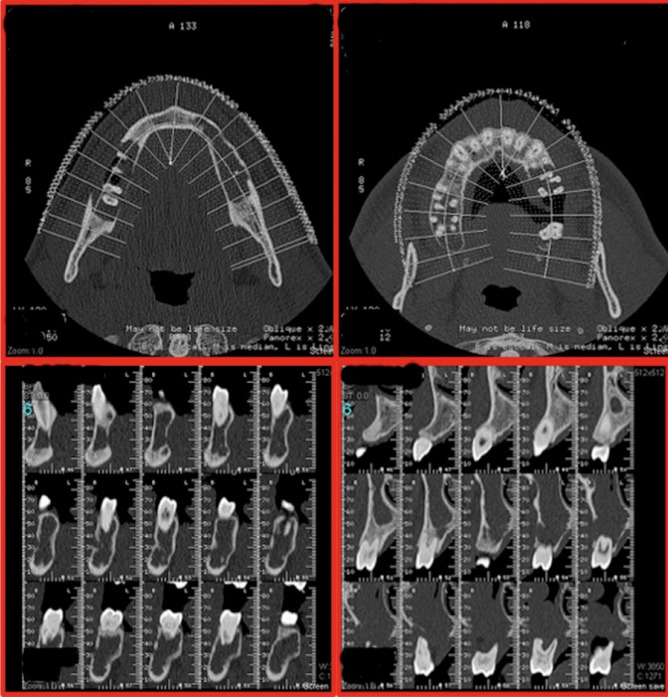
CT scan examination that confirms the presence of osteolytic lesions.
